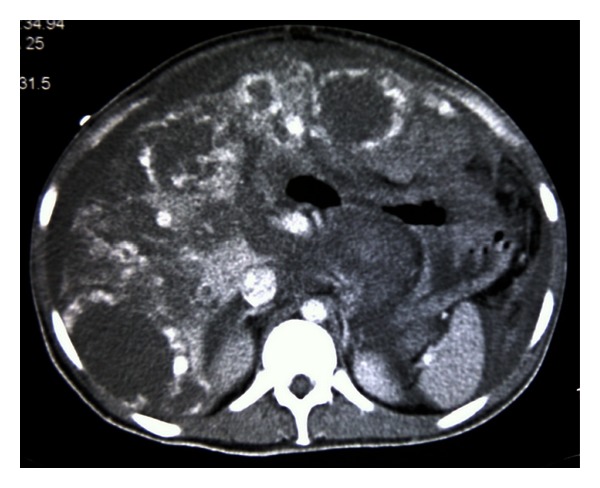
CT abdomen after development of hemorrhagic shock showed multiple heterogeneously enhancing masses in the liver with peripheral enhancement. Also, there is intraperitoneal fluid.
